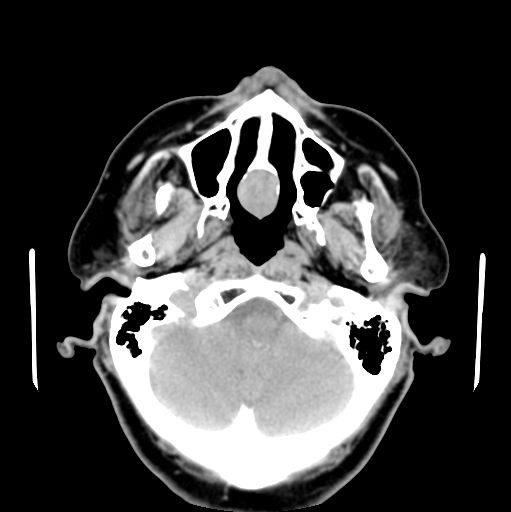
Axial non-contrast computed tomography scan demonstrating mass at posterior septum with extension into hard palate.
